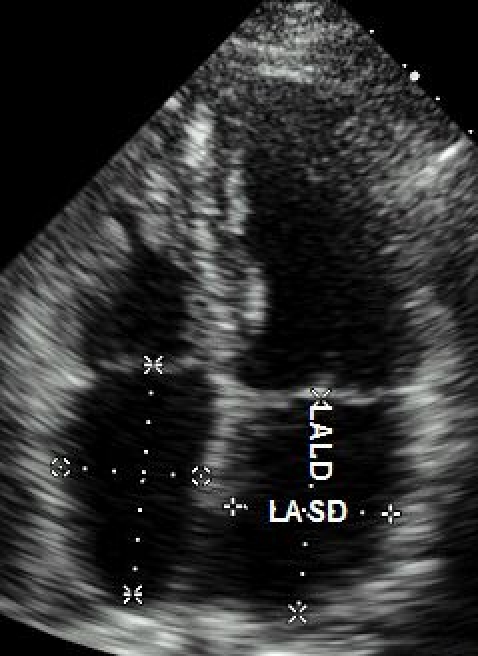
Measurement of left atrial size performed using apical four-chamber projection. LASD- left atrial short diameter; LALD-left atrial long diameter
